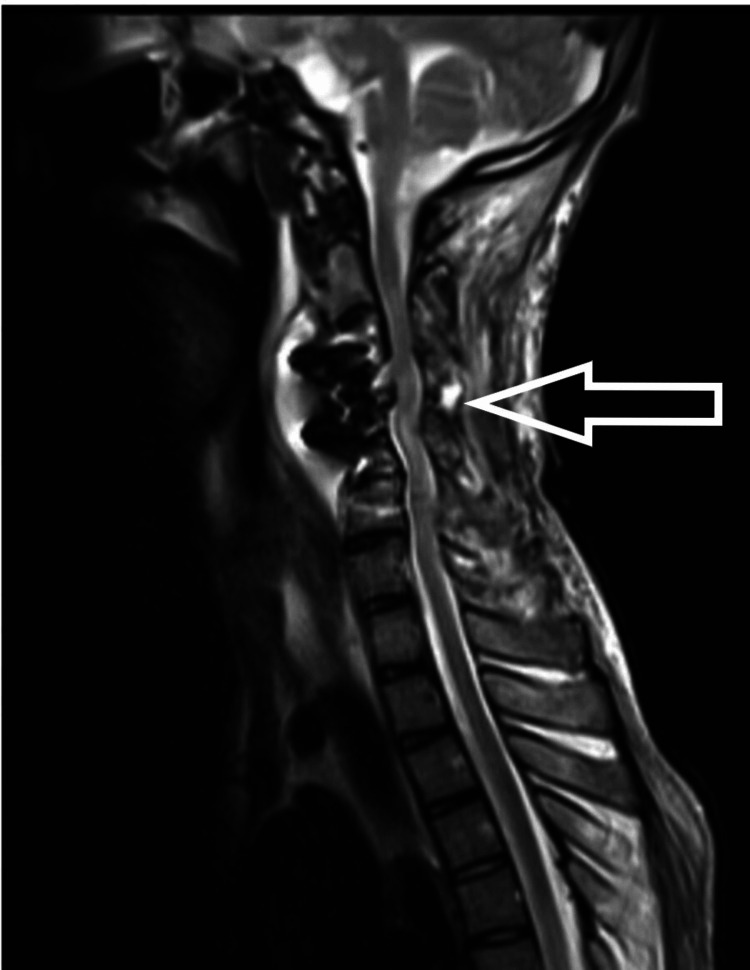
MRI showing diffuse leptomeningeal enhancement with pre-paravertebral collection (15 days after surgery) MRI: magnetic resonance imaging
